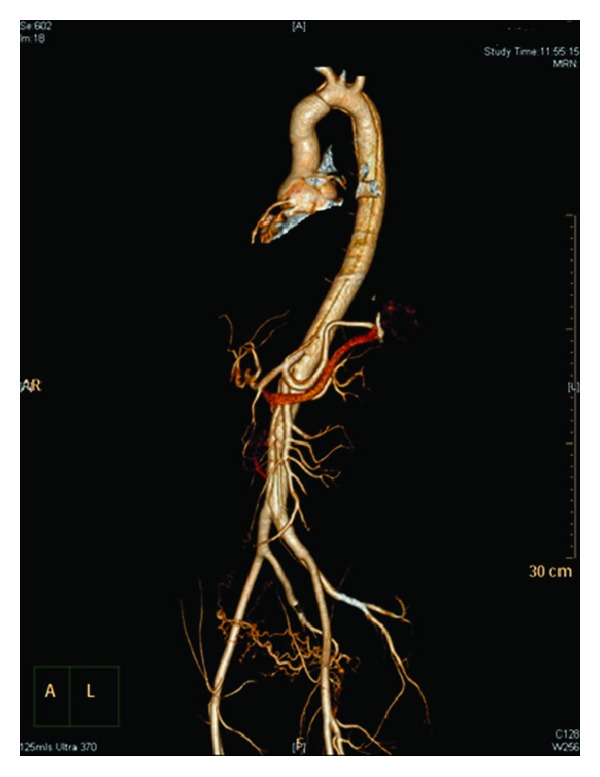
CT 3D reconstruction showing aortic dissection in the arch of the aorta extending along the descending thoracic aorta. Distally the dissection extends along the abdominal aorta up to the aortic bifurcations into the left common iliac artery.
